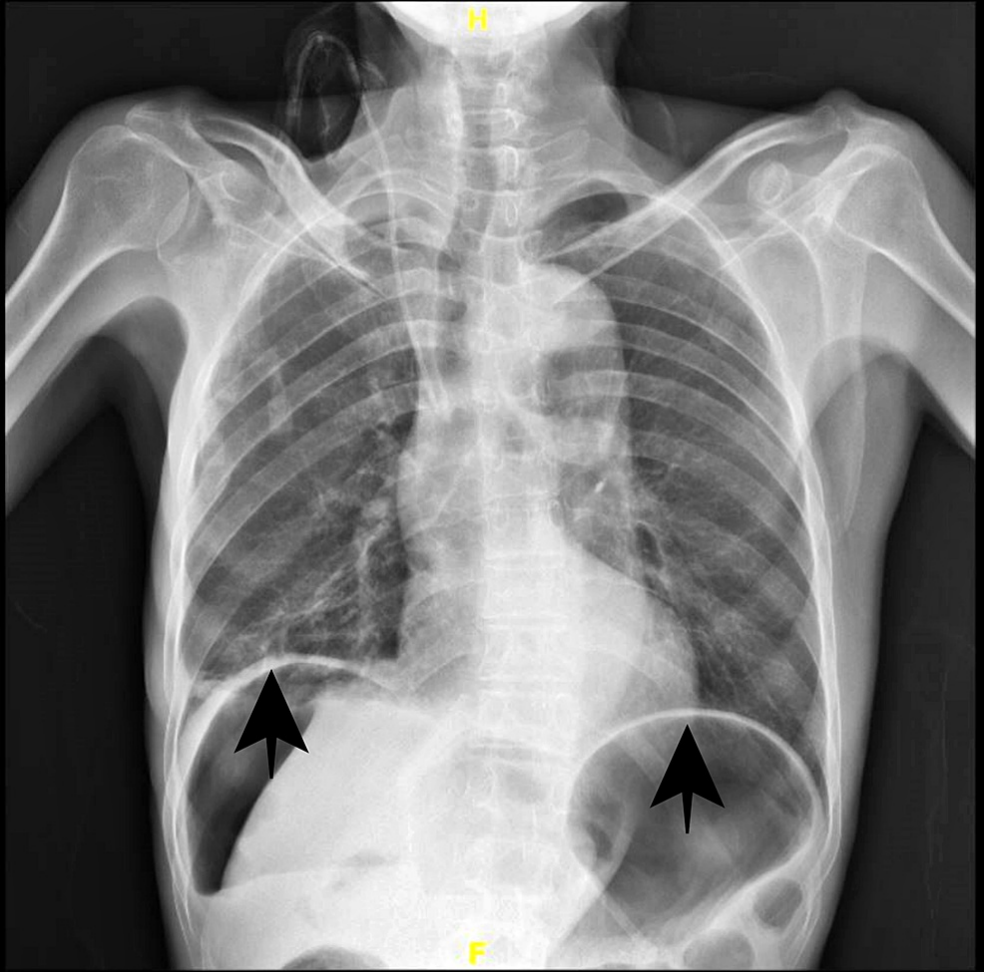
Chest X-ray showing air under the diaphragm (black arrows).
